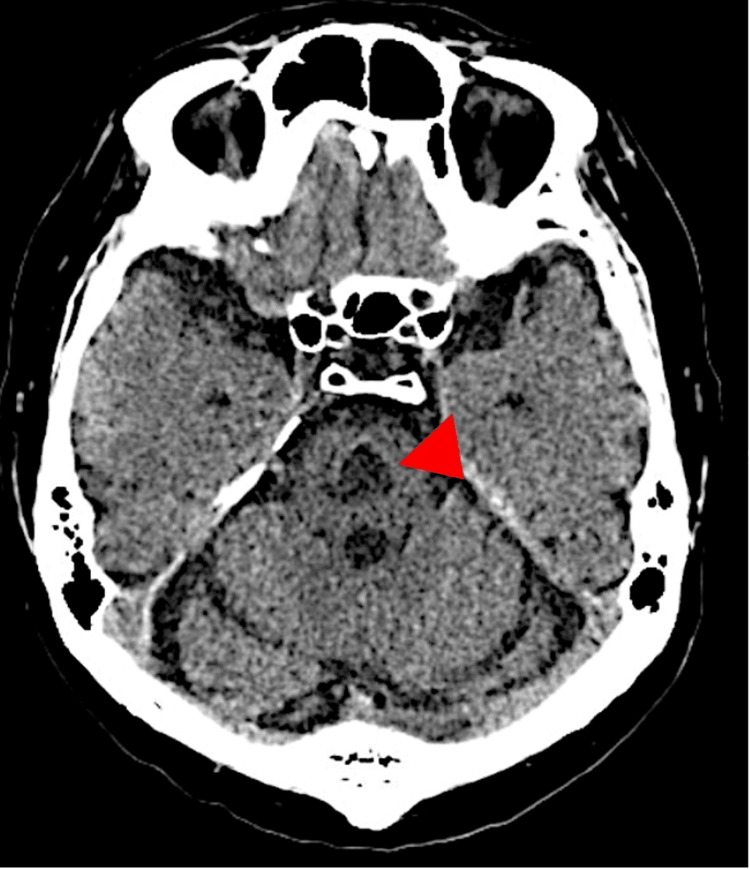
Axial non-contrast CT showing a hypodense lesion in the central pons (red arrowhead), consistent with ODS ODS, osmotic demyelination syndrome
